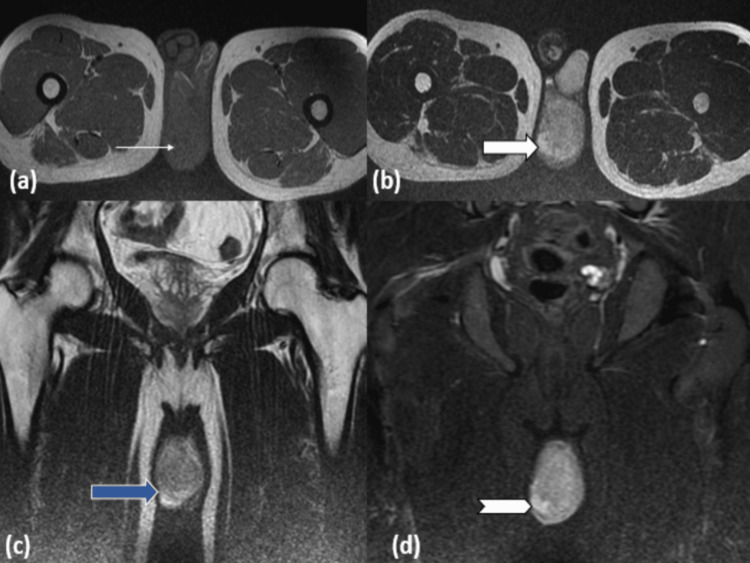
MRI axial T1-weighted image (a) at the level of testis showing iso to hypointense lesion in the right testis (line arrow). Axial (b) and coronal (c) T2-weighted image at the level of testis showing heterogeneously hyperintense lesion in the right testis with cystic spaces (block and blue arrow). Coronal STIR image (d) showing heterogeneously hyperintense lesion in the right testis (chevron arrow). STIR - short tau inversion recovery.
